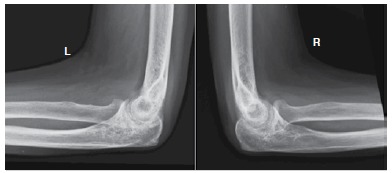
Radiographic alteration grade 3. Alterations on the articular surface can be seen, but the shape of the radial head is maintained and there are no loose bodies.
